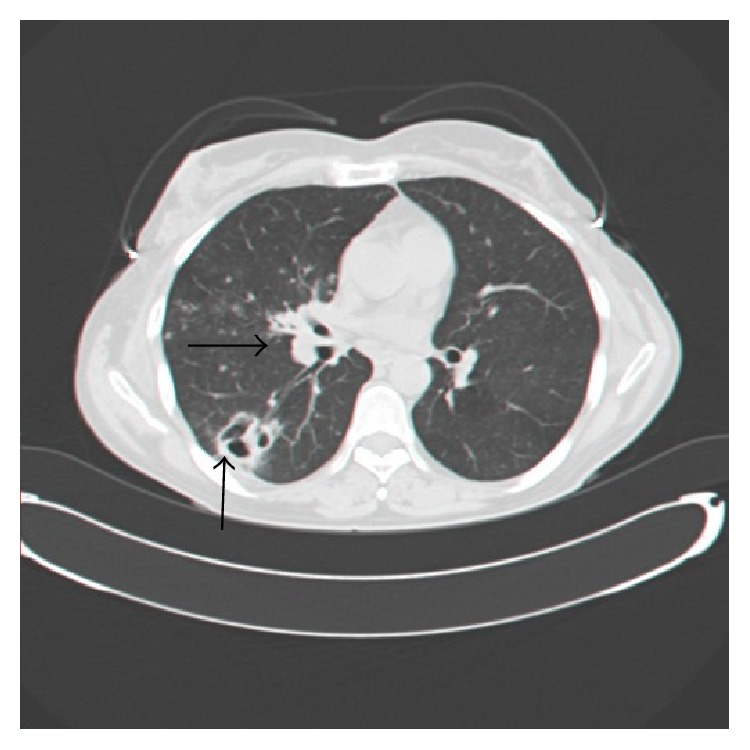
CT scan of the lung, axial view: the horizontal arrow is pointing toward nocardial mass in the right lower lob, while the longitudinal arrow is pointing toward nocardial cavitary lesion in the same lobe.
